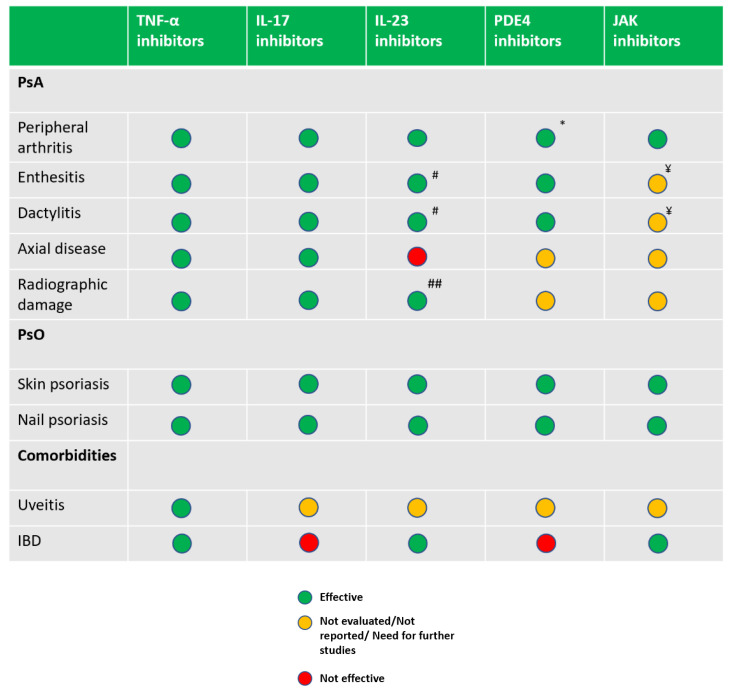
Efficacy of recently introduced therapeutic principles for PsA based on randomised controlled trials stratified for disease domain. ^#^ Risankizumab, no significant difference versus placebo in improving dactylitis and enthesitis [66]. ^##^ Ustekinumab, an anti-IL 12/23 p40 monoclonal antibody, inhibits radiographic progression in patients with active PsA [67]. Guselkumab, an anti-IL23 p19 monoclonal antibody, administered every 8 weeks, resulted in a non-significant decrease in radiographic progression compared with placebo, while Guselkumab every 4 weeks demonstrated significantly less progression of structural damage [68]. * Apremilast is not statistically different compared to placebo in the achievement of ACR70 [69]. ^¥^ With tofacitinib, the changes in scores from baseline with respect to enthesitis and dactylitis could not be declared statistically significant compared to placebo [70].
